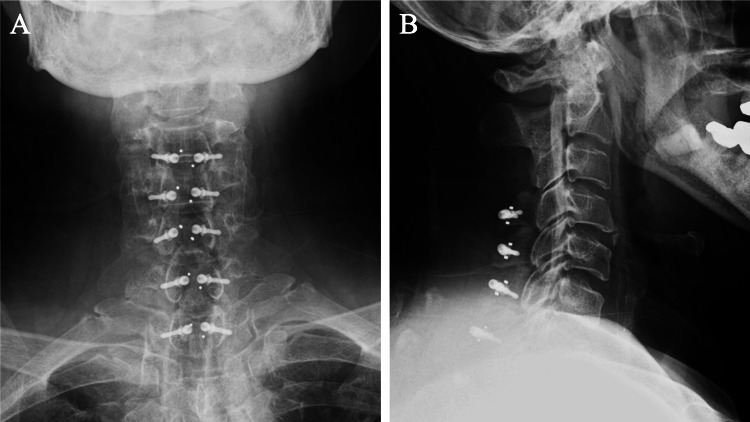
Postoperative radiograph of first operation (C2-Th2 decompression surgery, A: anteroposterior, B: lateral)
